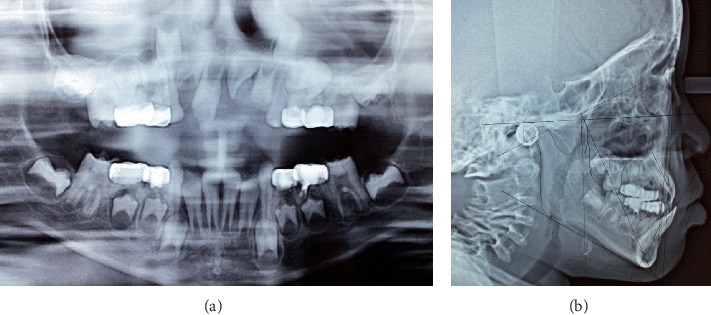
(a) Radiographic view and (b) Ricketts cephalometric analysis (June 2017).
